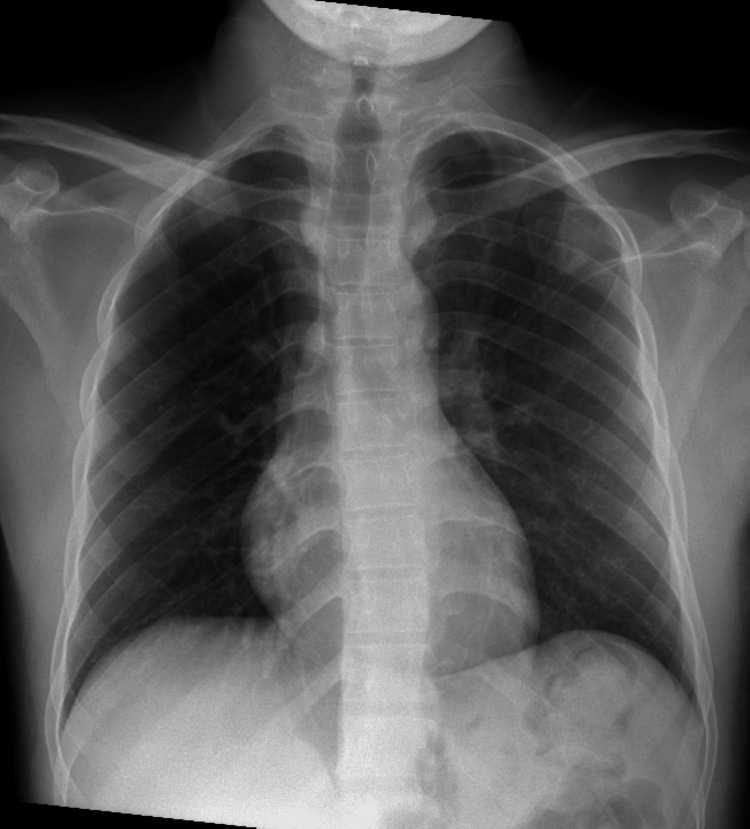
Chest X-ray on admission was normal
